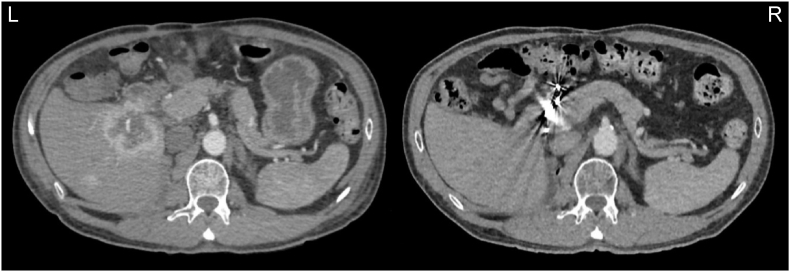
Abdominal CT before combination therapy with nivolumab plus ipilimumab (left) and after 6 months (right), showing significant reduction of liver metastases.
